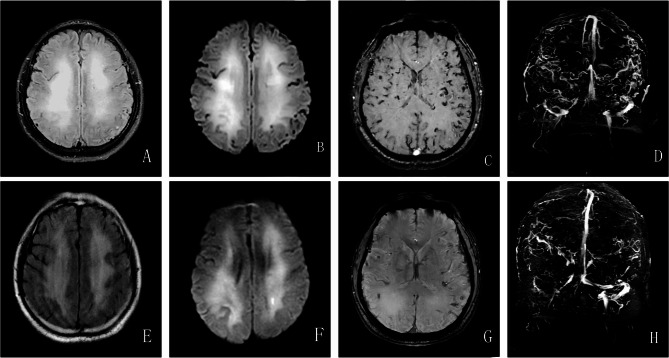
Brain MRI scan. T2-weighted FLAIR (A) and DWI (B) sequences of brain MRI scans reveal abnormal white matter signals in bilateral corona radiata areas. SWI (C) and MRV (D) sequences of brain MRI scans reveal extensive tortuous dilatation of intracranial veins. After DAVF embolization, T2-weighted FLAIR (E) and DWI (F) brain MRI scans show improvement in white matter lesions. SWI (G) and MRV (H) sequences of brain MRI show a significant reduction in multiple vascular shadows on the brain surface and subcortex. Abbreviations: MRI = magnetic resonance imaging; FLAIR = fluid attenuation inversion recovery; DWI = diffusion-weighted imaging; SWI = susceptibility-weighted imaging; MRV = magnetic resonance venography; DAVF = dural arteriovenous fistula
